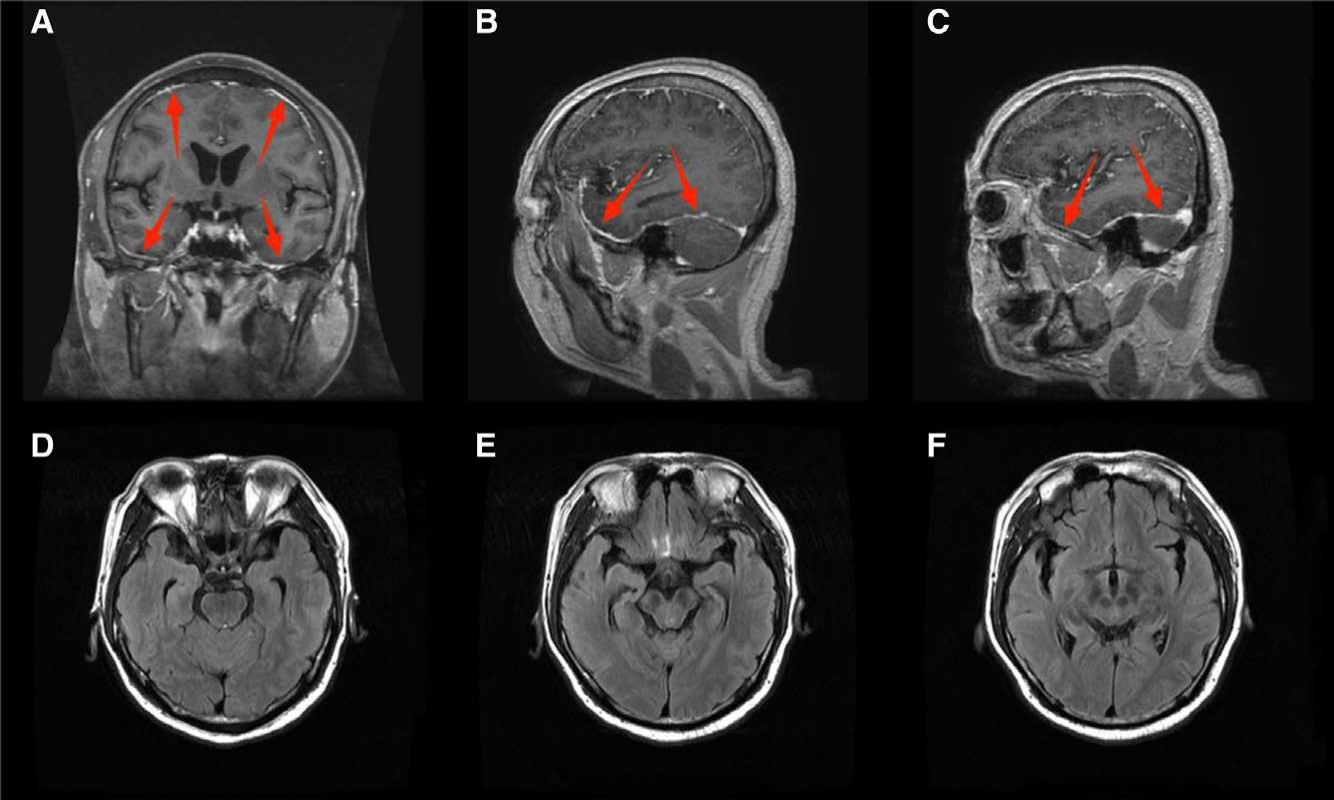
Brain MRI. (A–C) Sagittal and coronal T1-weighted imaging (T1WI) with fat suppression: the surface of the bilateral tentorium cerebelli and dura mater shows mild thickening and rough edges; the areas pointed by red arrows are the thickened and rough parts; (D–F) Axial T2-weighted fluid-attenuated inversion recovery (T2FLAIR) images: no abnormal signals were observed in the bilateral temporal lobes, hippocampus, and insular cortex. MRI = magnetic resonance imaging.
